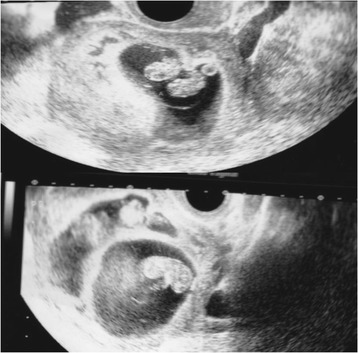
Ultrasound image of a dichorionic triamniotic triplet in the seventh week of pregnancy
